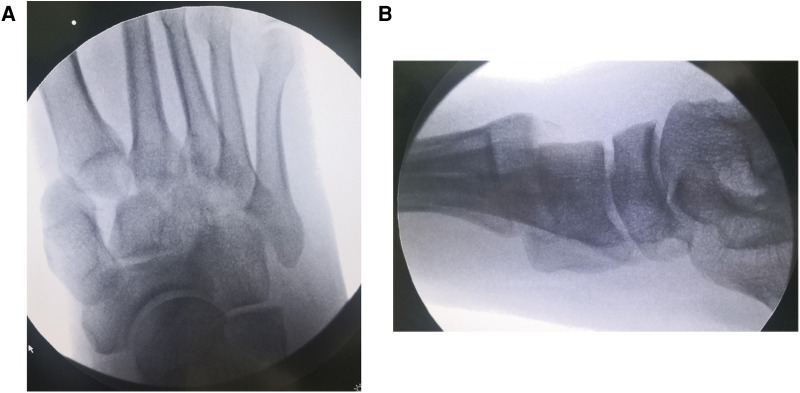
Anteroposterior and lateral radiographs of the patient's injured foot on admission, showing complete dislocation of the metatarsophalangeal joint. (A) Anteroposterior (B) Lateral.
